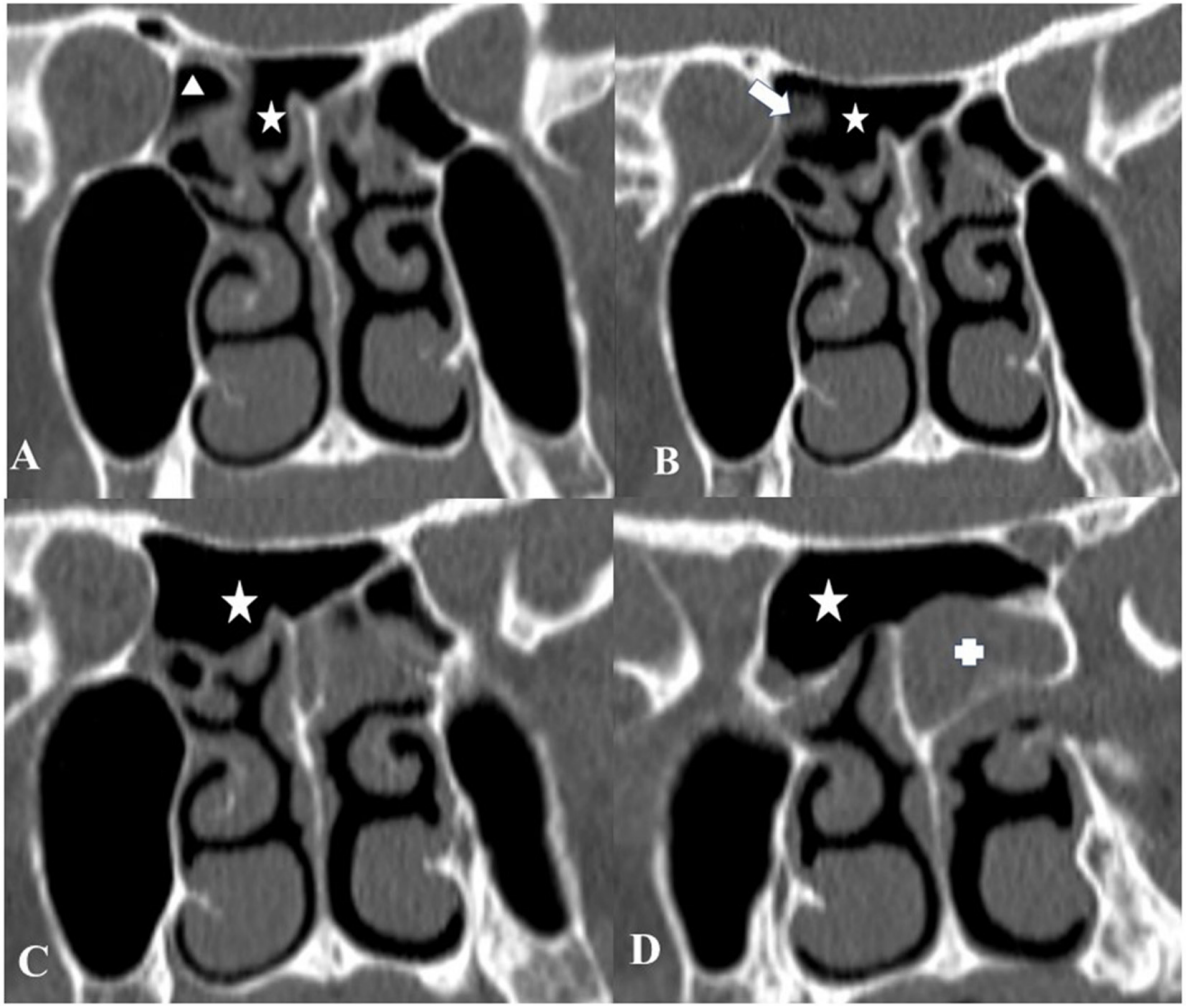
Coronal CT scans of the paranasal sinuses show an SSC (star) on consecutive sections. A right posterior ethmoid cell (triangle) is seen medial to the orbit (A). A posterior wall of this cell (arrow) is anterior to the optic canal (B). Note that an SSC (star) is extending beyond this posterior ethmoidal cell laterally (C) and attaches to optic nerves bilaterally (D).
